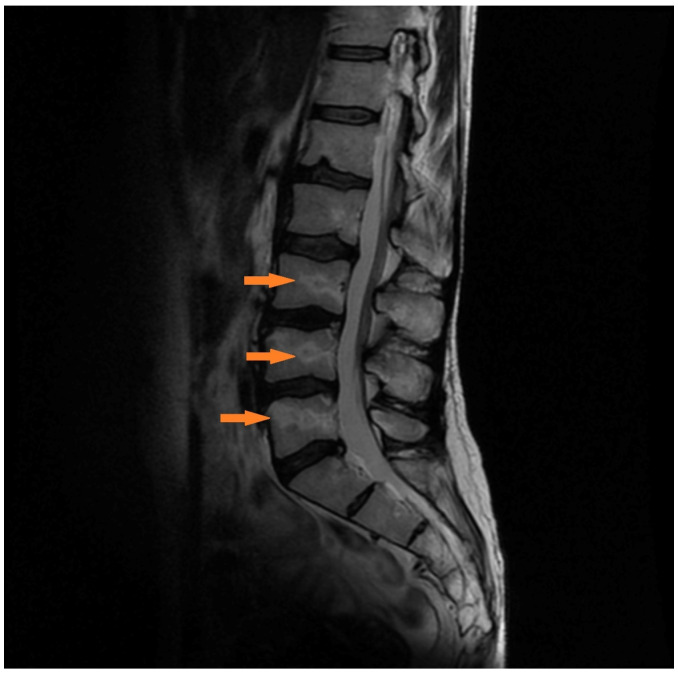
Hyperintense geographic edema in the lower lumbar on T2-weighted sagittal MR images, representing beginning bone necrosis. Delineation of the necrosis towards the vertebral body (orange arrow).
